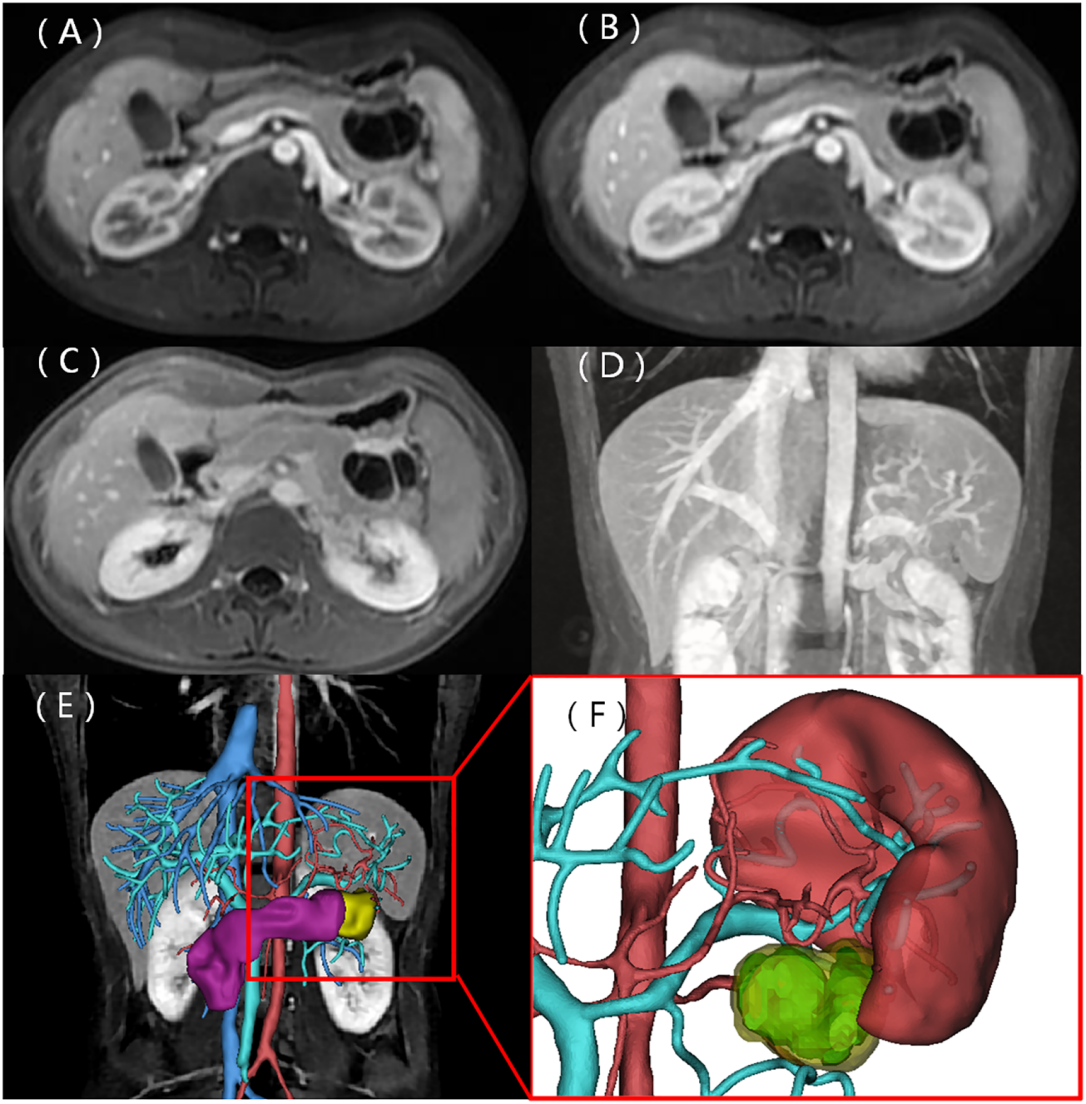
Contrast-enhanced MRI scans. The solid components and septa were slightly enhanced in the arterial phase, with delayed enhancement observed in the venous and delayed phases (A–C). The coronal MIP image displays enlargement of the portal vein and tortuous dilation of blood vessels in the splenic hilum (D). (E) Preoperative three-dimensional MRI of the tumor and adjacent structures. (F) Cystic (light blue) and solid (yellow) components of the tumor.
